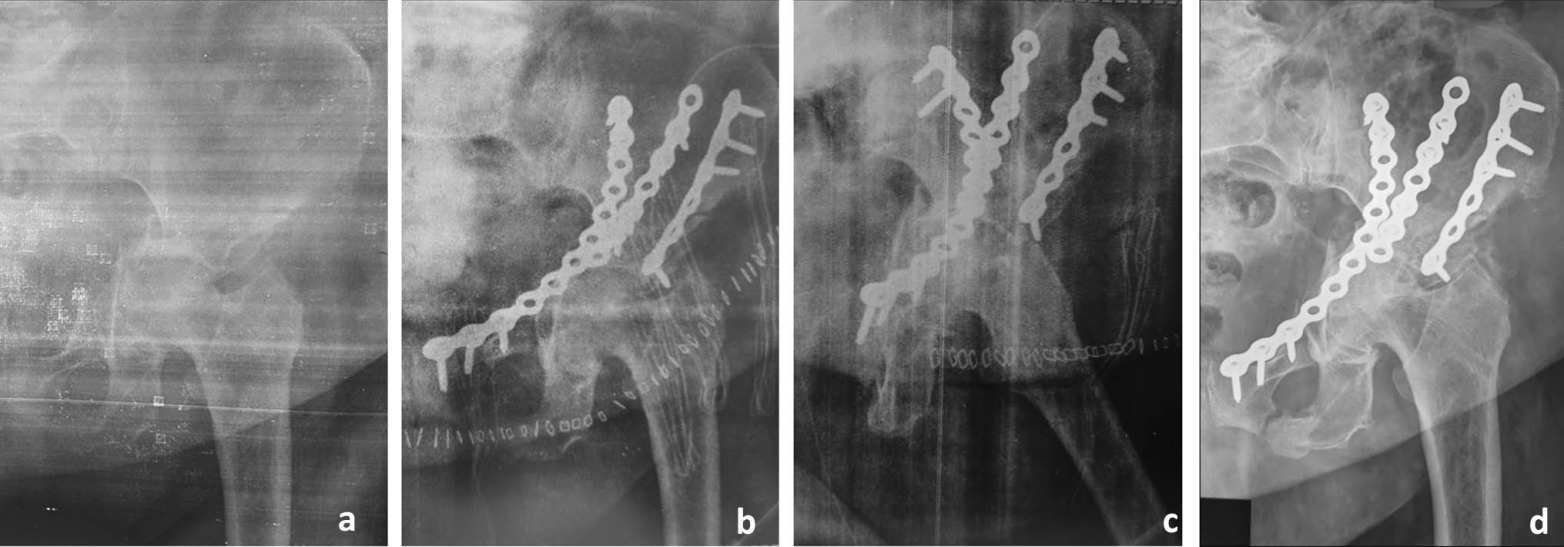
a Anteroposterior X-ray view of a fragility both column fracture occurred in an 84-year-old female. Please note the degree of fracture fragmentation and the involvement of the quadrilateral plate. b and c Anteroposterior and oblique postoperative X-rays. Because of the patient started to complain respiratory failure during the procedure, a non-anatomic reduction of the quadrilateral plate was accepted. d Anteroposterior X-ray at 1 month of the surgery showing reduction loosening of the quadrilateral plate and femoral head medialization
